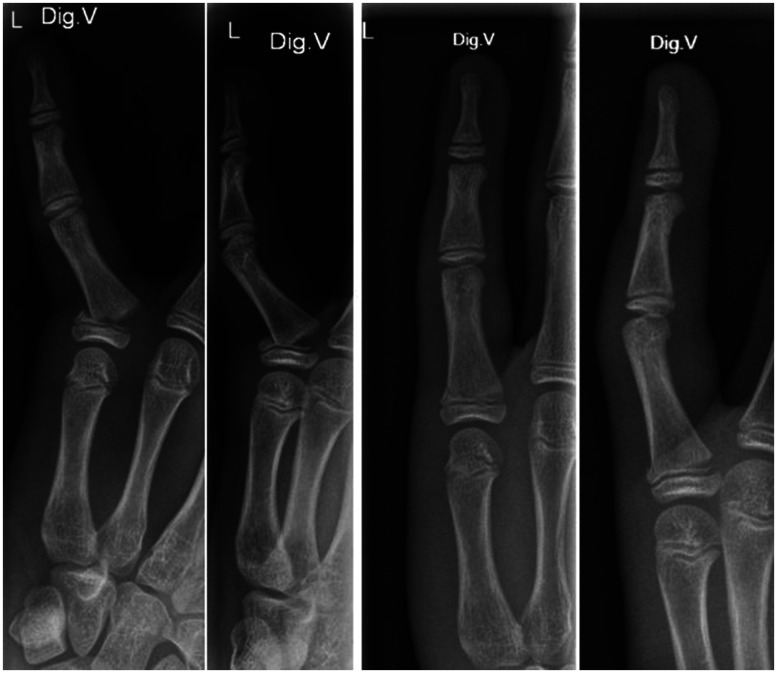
Example of a juxta-epiphyseal fracture of the proximal phalanx of the little finger treated with buddy taping. Posteroanterior and lateral radiographs at initial presentation and after 21 days.
